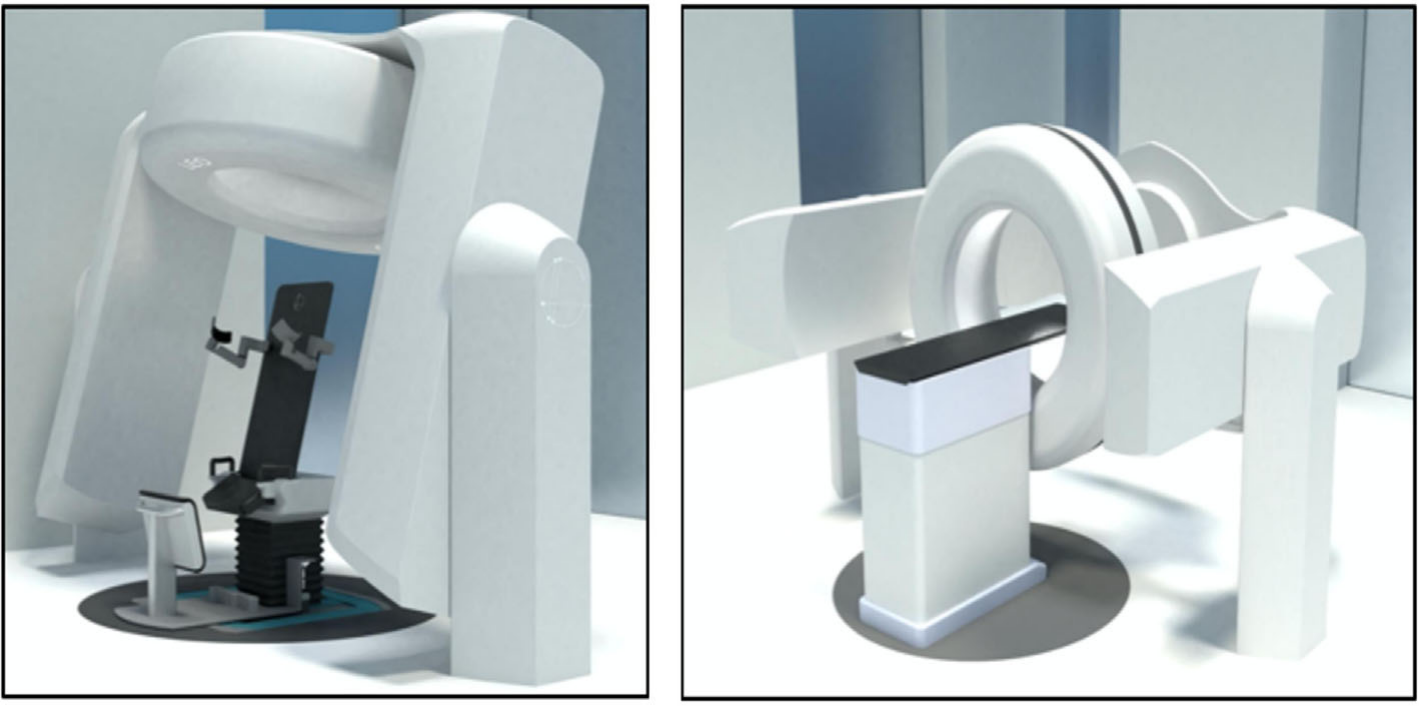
The left panel shows an upright Dual Energy CT scanner together with the upright patient positioner for upright scanning and treatments that is under development. The right panels show the CT scanner integrated with a CT gurney for CT scanning in the lying down position (courtesy LEO Cancer Care Ltd).
